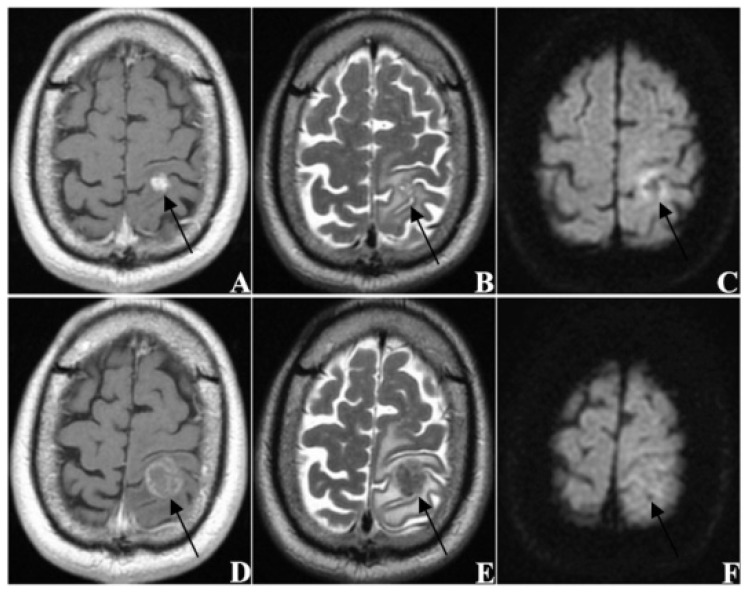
A sixty-year-old male patient (KPS = 70) with brain metastases (*n* = 1) from lung cancer. The primary tumor recurred after surgery with mediastinal lymph node metastasis. (A–C) re MRI images taken before SRS treatment, and (D–F) are MRI images taken at follow-up 60 days after treatment ((A,D) from CE-T1WI; (B,E) from T2WI; (C,F) from DWI). The tumor was located in the left frontoparietal junction and showed homogeneous enhancement. The EI was 4.38. After treatment, the tumor was obviously enlarged and the edema was aggravated, thus this patient was classified as PD.
